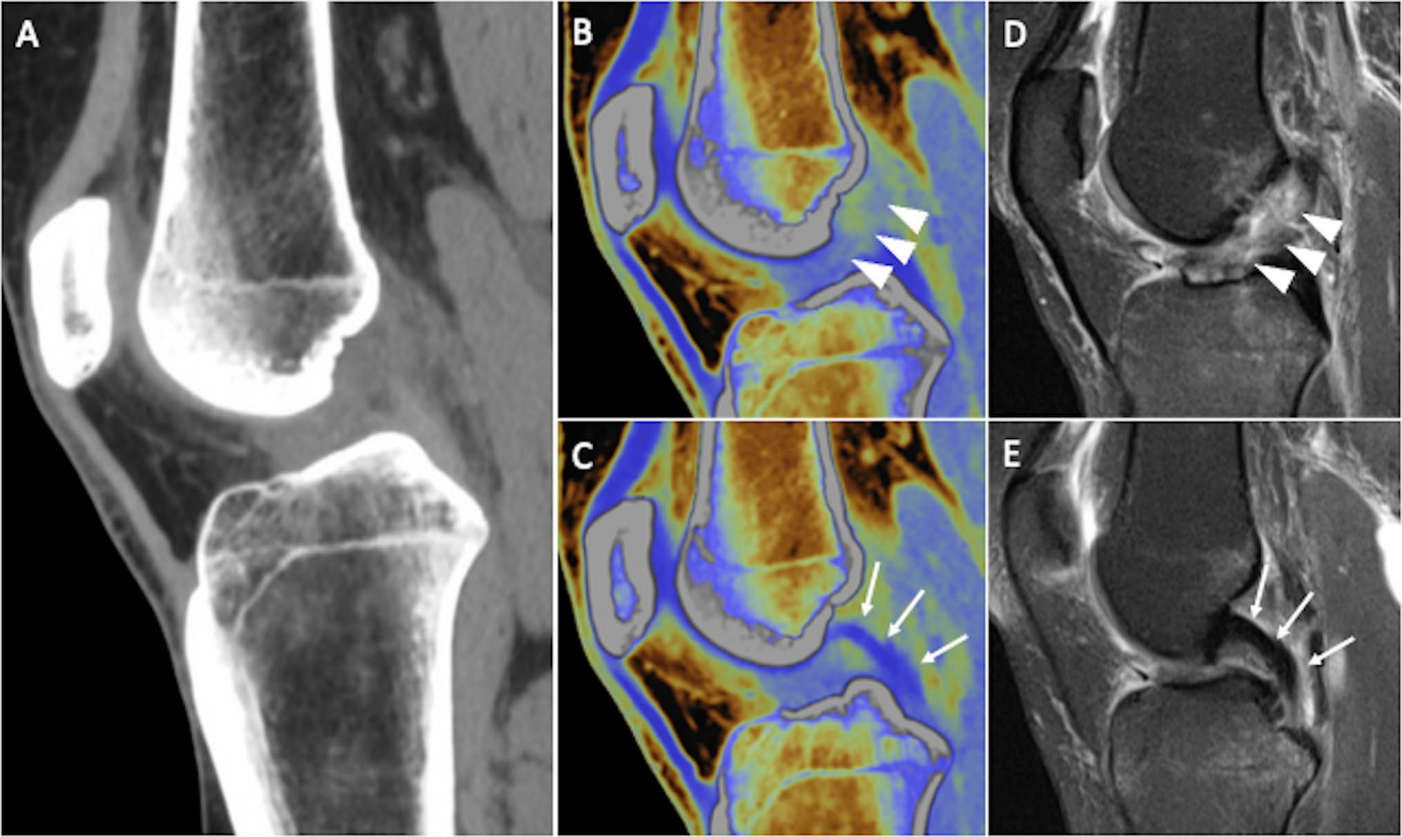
Anterior cruciate ligament tear in a 42-year-old man after knee sprain. Sagittal reformat of standard knee CT (a) is not helpful for describing clearly cruciate ligaments status. Sagittal virtual noncalcium DECT images allow the identification of anterior cruciate ligament injury (b, arrowheads), with normal appearance of posterior cruciate ligament (c, arrows), as proven by the corresponding sagittal fat-suppressed proton density weighted MRI images (d and e, respectively)
